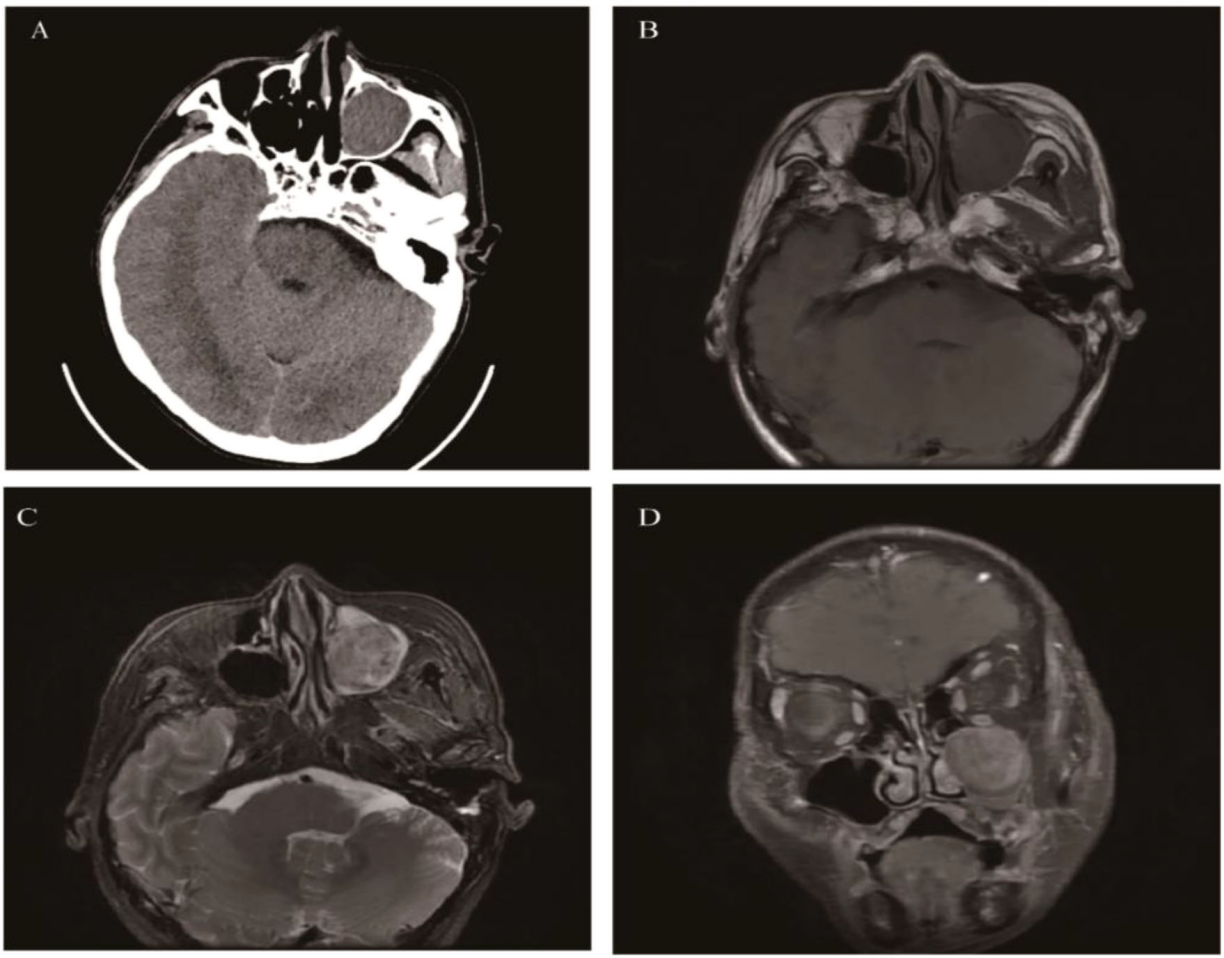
Schwannoma: Axial CT showed a well-defined, round soft tissue mass in the left maxillary sinus causing slight sinus cavity enlargement (A). Axial T1WI (B) and Axial T2WI (C) exhibited isointense and heterogeneous hyperintense signal intensity tissue which showed moderate enhancement on coronal contrast-enhanced images (D).
